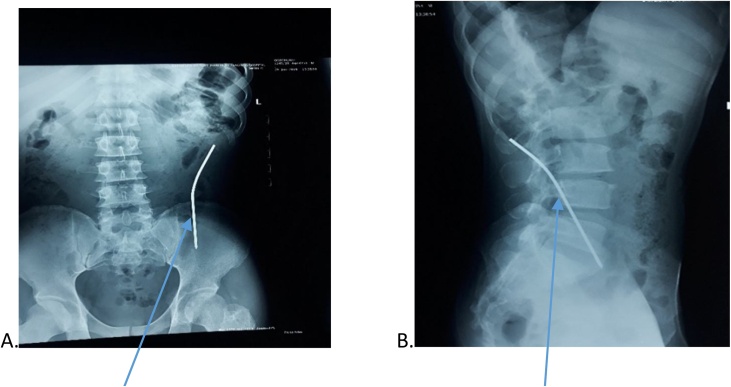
Plain abdominal radiographs showing migrated pin into abdominal cavity A) anteroposterior view. B) Lateral view.
